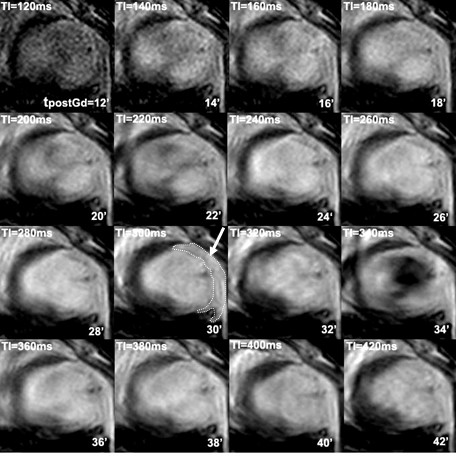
Short-axis cardiovascular MR images corresponding to each inversion time (TI) following Gd-DTPA injection (the dotted line shows the hyperenhanced area corresponding to the infarcted myocardium at 30 minutes post-Gd-DOTA injection).
